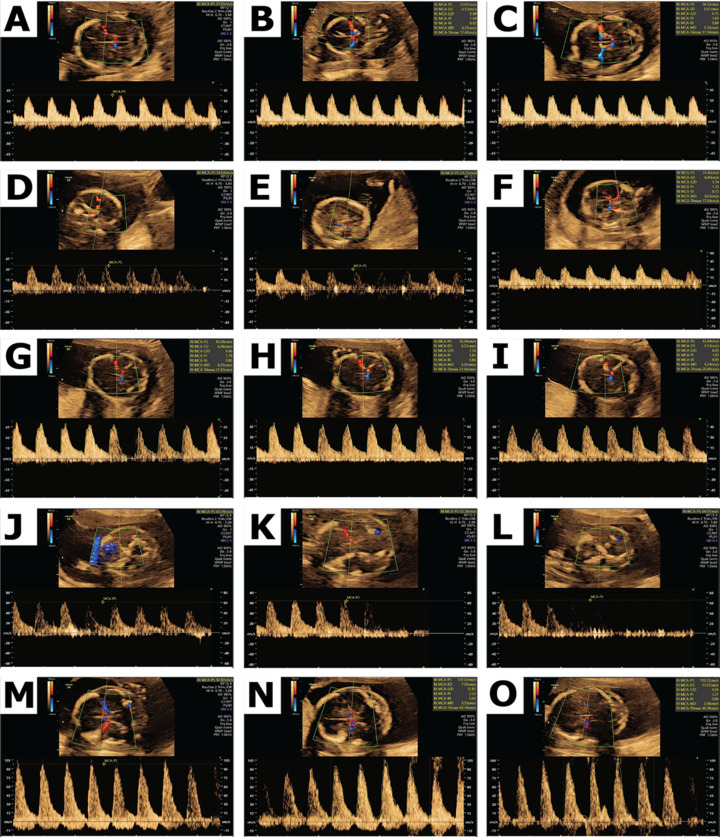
representative Doppler images demonstrating fetal middle cerebral artery peak systolic velocity (MCA-PSV) measurements across gestation: A-C) 18+2 weeks, MCA-PSV 34.78 cm/s (1.55 MoM); D-F) 19+2 weeks, MCA-PSV 34.69 cm/s (1.45 MoM); G-I) 20+2 weeks, MCA-PSV 43.49 cm/s (1.7 MoM); J-L) 21+3 weeks, MCA-PSV 62.98 cm/s (2.3 MoM); M-O) 24+0 weeks, MCA-PSV 99.33 cm/s (3.3 MoM)
